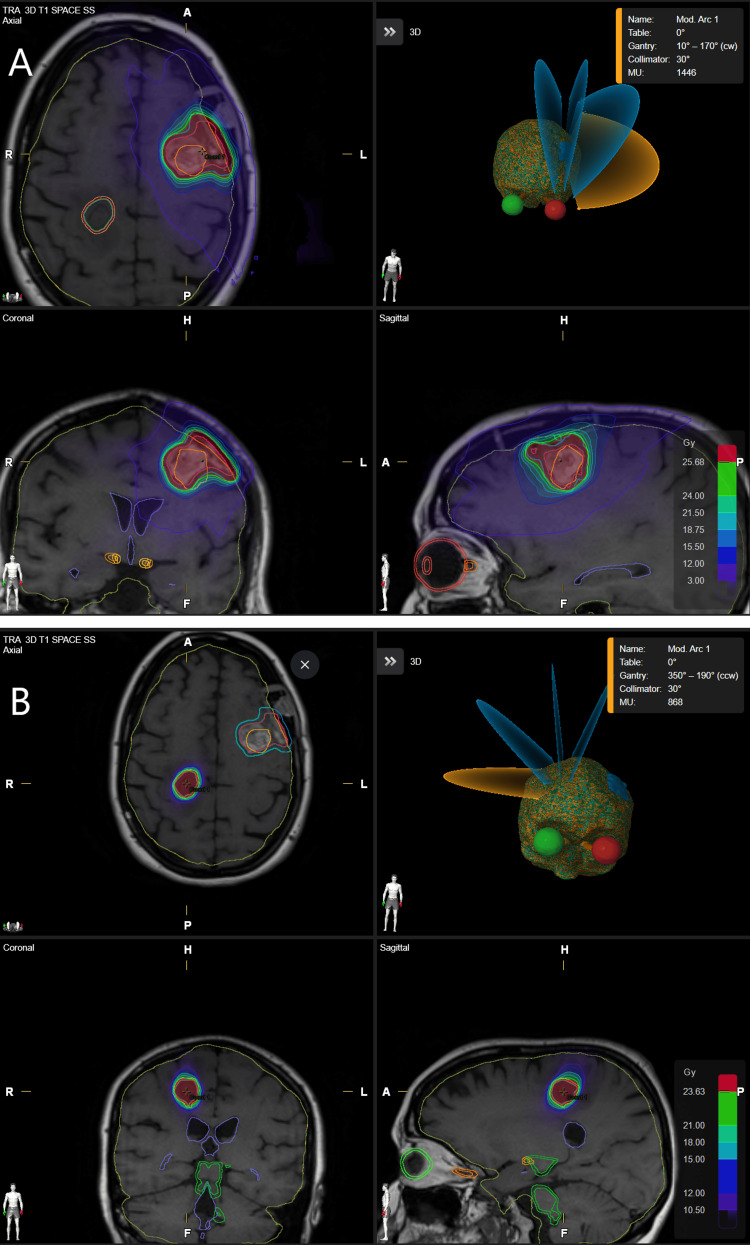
Dose distributions for a typical cSRS patient with a left-sided cavity and a right-sided intact metastasis, treated concurrently. (A) The left-sided cavity with the planning target volume (PTV) (blue) grown with a 2 mm margin from the clinical target volume (CTV) (red). The orange contour indicates the position of the lesion on the pre-operative MRI scans. The prescription isodose of 24 Gy in three fractions is shown in green. (B) The right-sided intact met with the PTV (red), grown with a 1 mm margin from the CTV (blue). The prescription isodose of 21 Gy in one fraction is shown in green. The intact met was treated concurrently with the cavity. cSRS: cavity stereotactic radiosurgery.
